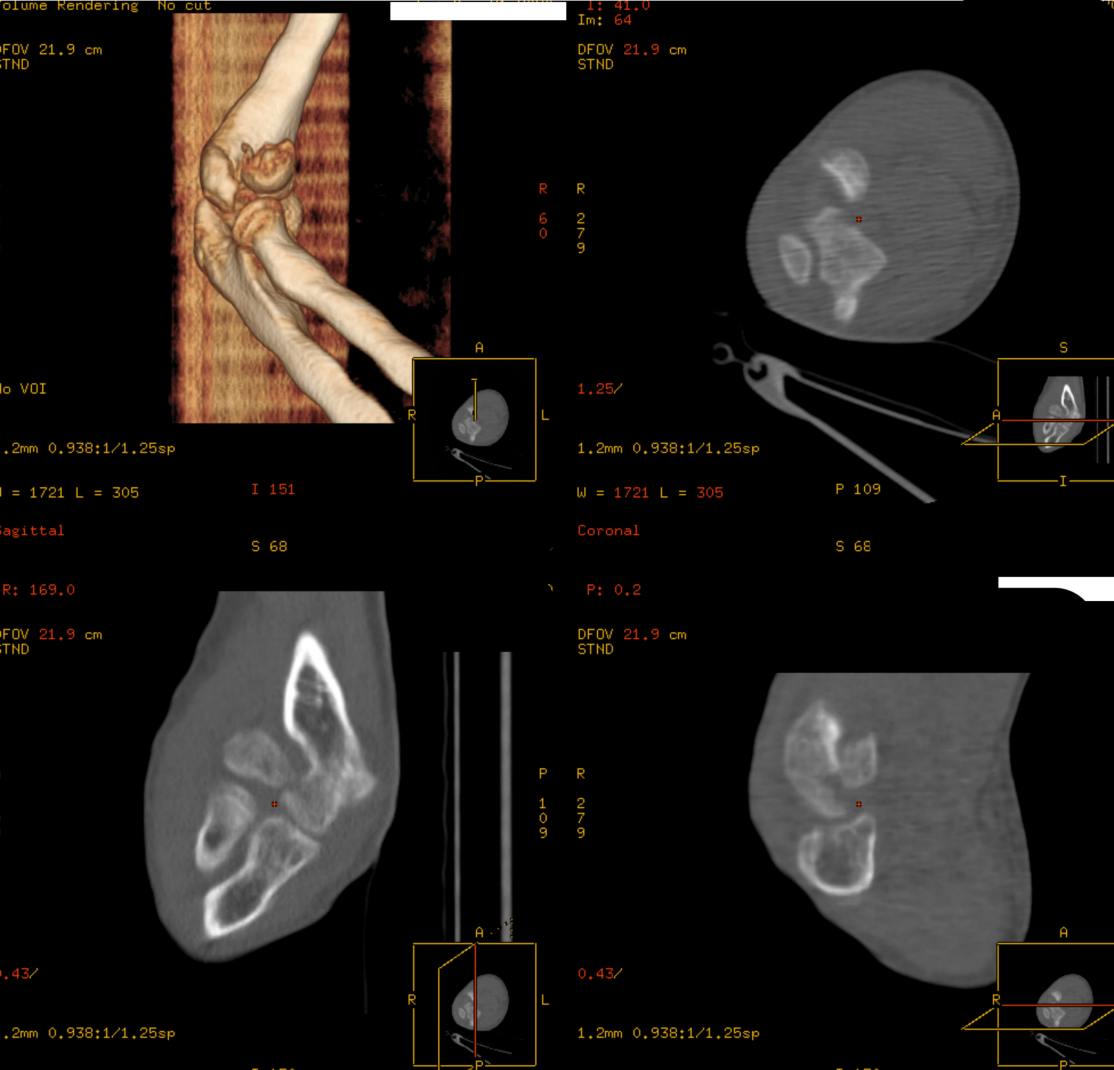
Preoperative CT assessment of the capitellar fracture.
